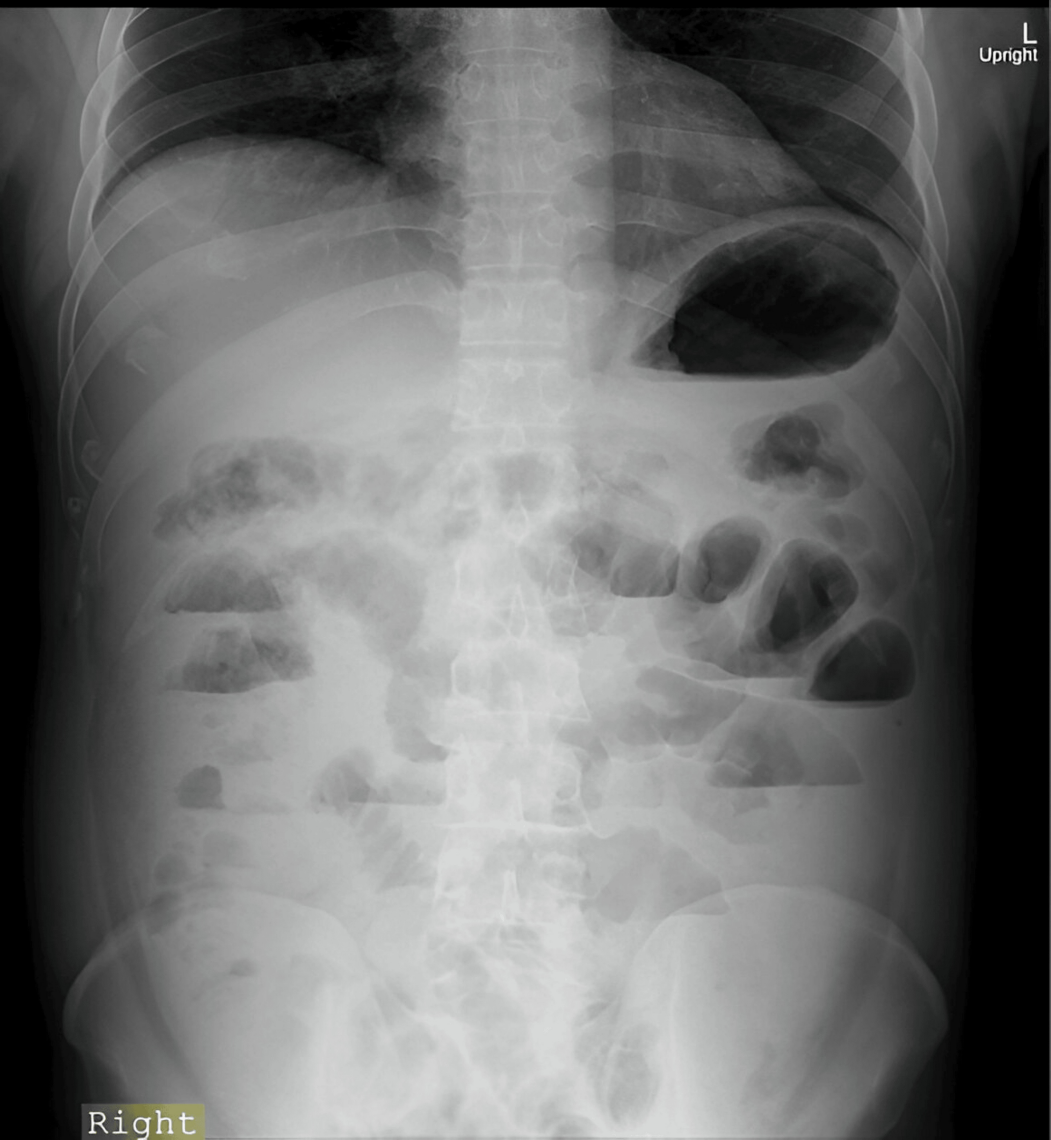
Abdominal X-ray showing multiple air fluid level
